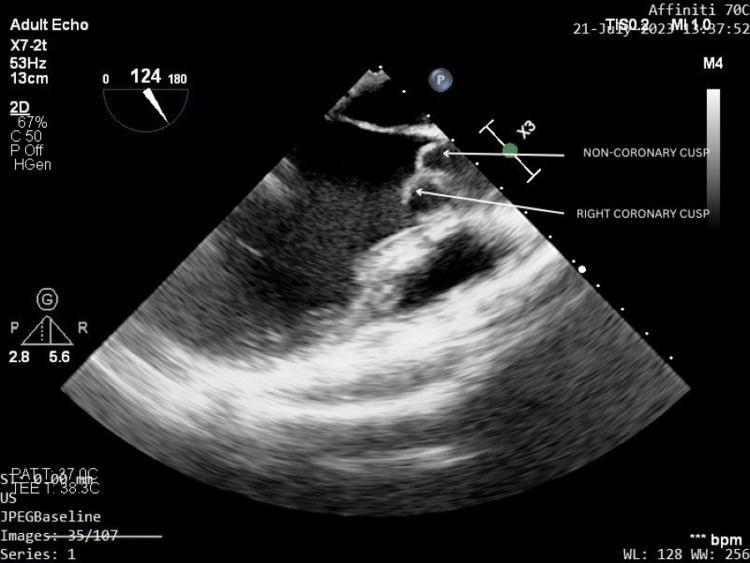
Prolapsed non-coronary and right-coronary cusps of the aortic valve visible on a 120-degree long-axis view of transesophageal echocardiography. The above image has been taken by the authors.
